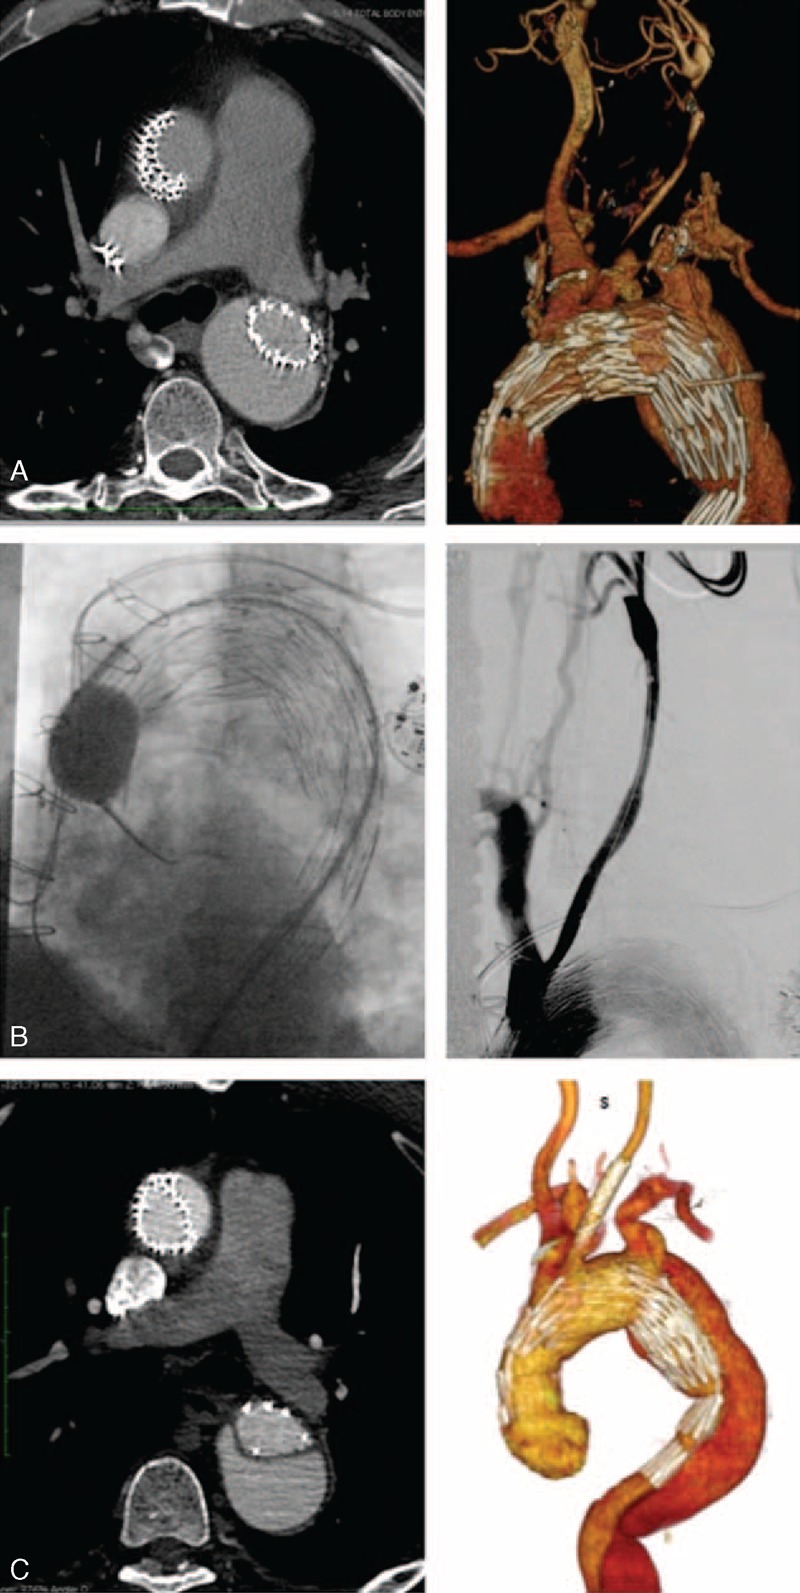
(A) Angio-CT documenting the proximal infolding of the endograft and the left common carotid dissection; (B) angioplasty of the proximal infolding and retrograde angiography of the dissected left common carotid; (C) angio-CT documenting the residual proximal leak and no residual dissection of the left common carotid. CT = computed tomography.
